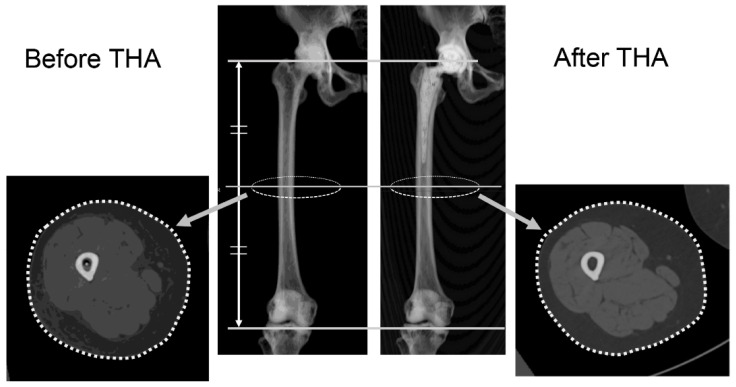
Measurement of thigh circumference and cross-sectional area using CT images before and after THA. After the femur was aligned to the radiographic coronal plane, the circumference and cross-sectional area of the thigh were measured on a plane perpendicular to the *z*-axis, which passes through the midpoint between the tip of the greater trochanter and the most distal end of the femoral condyles. CT, computed tomography; THA, total hip arthroplasty.
